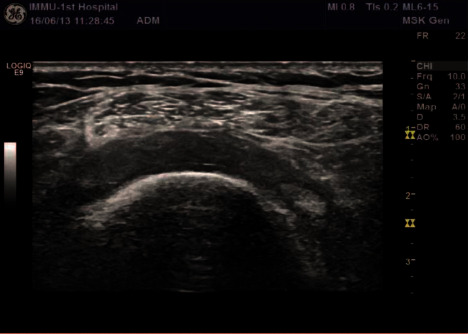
Two-dimensional ultrasound normal supraspinatus tendon.
